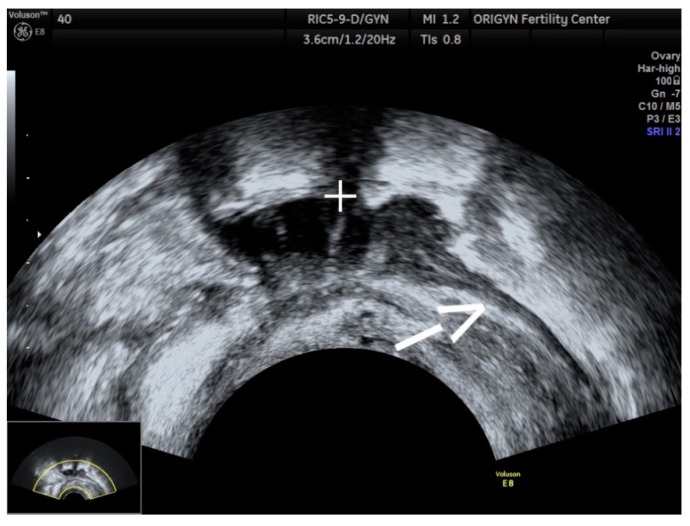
Ultrasound image showing a DIE nodule (+) with progressive narrowing, like a “tail” (white arrow), also known as a “comet” sign.
